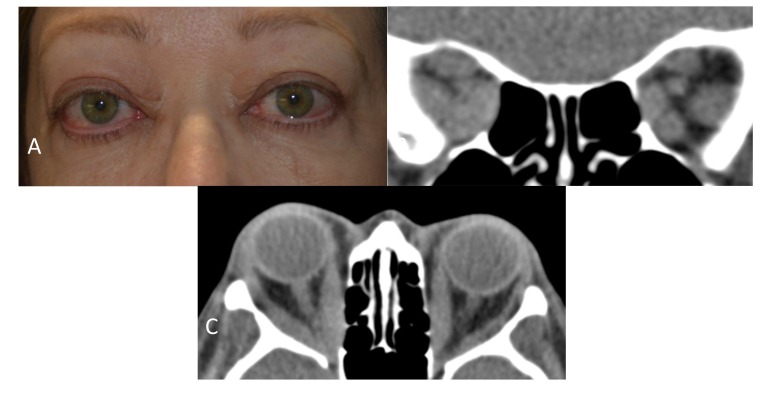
A: Clinical photograph of a case with severe, active thyroid eye disease with exophthalmos, congestive orbitopathy, and compressive optic neuropathy. B: A Computed Tomography (CT) coronal section through the orbital apex shows enlargement of the extraocular muscles and compression of the optic nerve on the right side. C: A CT axial section demonstrates marked enlargement of the extraocular muscles with right optic nerve compression at the orbital apex.
